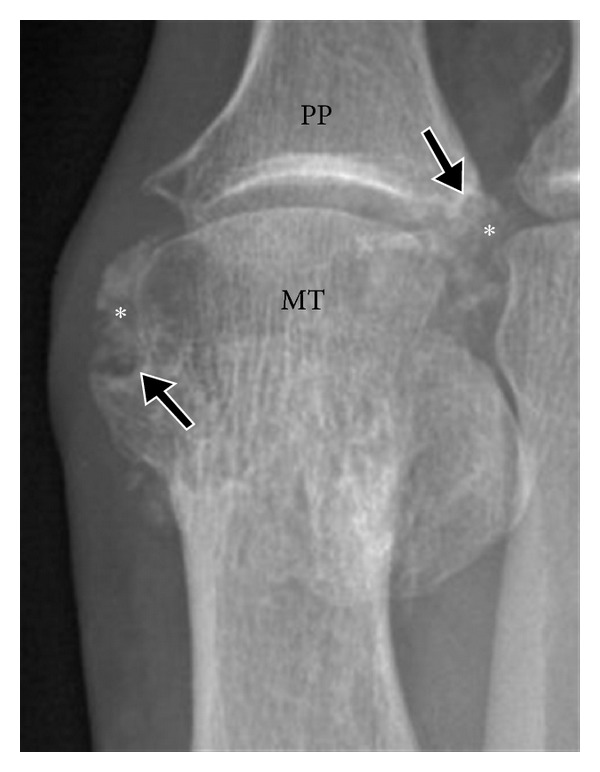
Tophaceous gout involving the 1st MTP joint. Anteroposterior radiograph shows a calcified soft tissue tophus (asterisk) with adjacent erosions (arrow). MT: first metatarsal head; PP: proximal phalanx. Possible associated calcium pyrophosphate or hydroxyapatite deposition must be considered.
